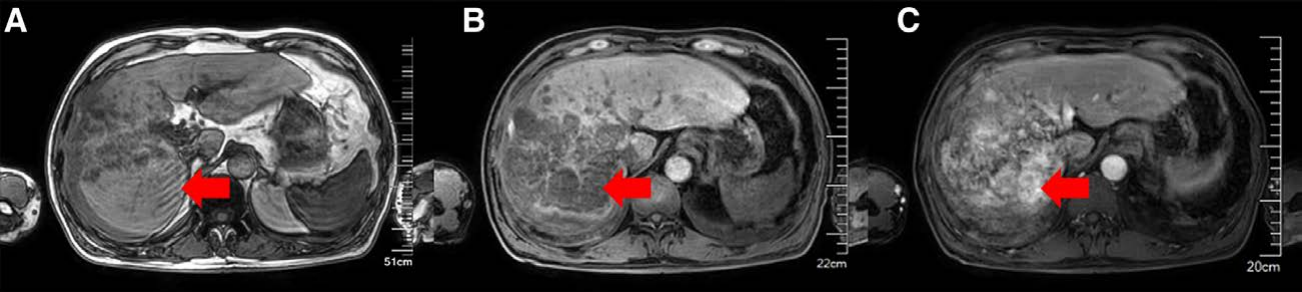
MRI examination on November 17 showed that the surface of the liver was rough with multifocal masses of T1 and T2 signal intensities. The right lobe of the liver exhibited enhancement (A), while the venous phase showed uneven enhancement, which was higher than that in the liver parenchyma (B). The arterial phase exhibited a tubular enhancement shadow proximal to the artery (C). MRI = magnetic resonance imaging.
